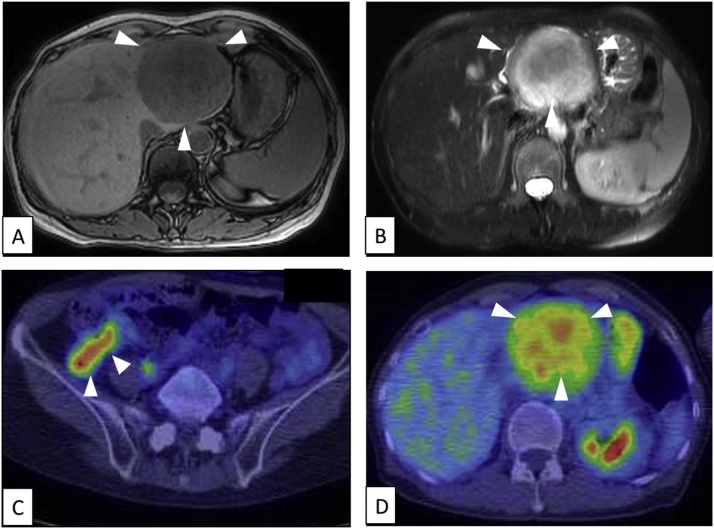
Preoperative MRI and FDG PET/CT scan findings. (A) T1-weighted MRI image showing low intensity. (B) T2-weighted MRI image showing heterogeneous high intensity. (C, D) FDG PET/CT scan image showing increased tracer accumulation in both the colon (SUVmax = 5.66) and liver (SUVmax = 5.37) lesions.
